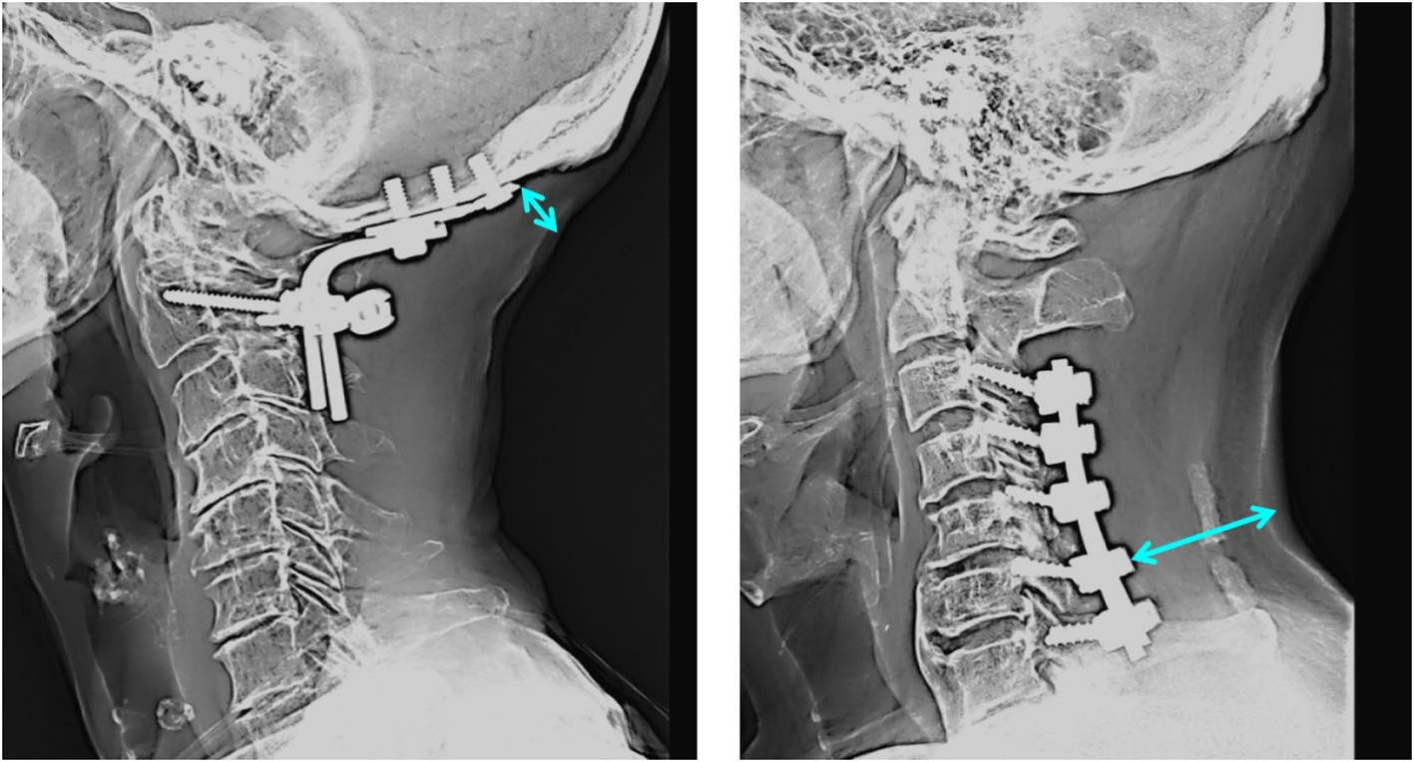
Left: Radiographic lateral image following occipito-C2 posterior instrumented fusion surgery. The soft tissues covering the metal implants placed on the occipital bone are relatively thin (bi-directional arrow). Right: Radiographic lateral image following sub-axial cervical spinal posterior instrumented fusion surgery. The soft tissues covering the metal implants are relatively thick (bi-directional arrow).
